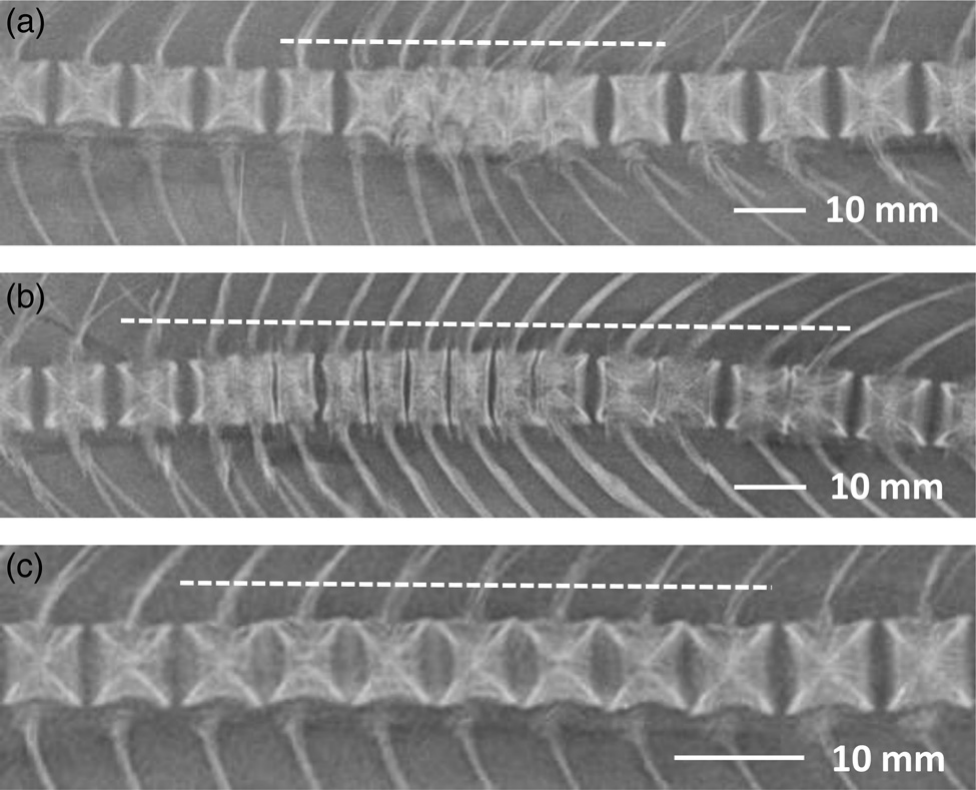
Radiography: detail of vertebral lesions. (a) Fused vertebrae, lesion located under the dorsal fin; (b) platyspondylia, located in caudal spine; (c) missing intervertebral space, lesion located in cranial spine. Dotted lines indicate the extent of the lesions.
